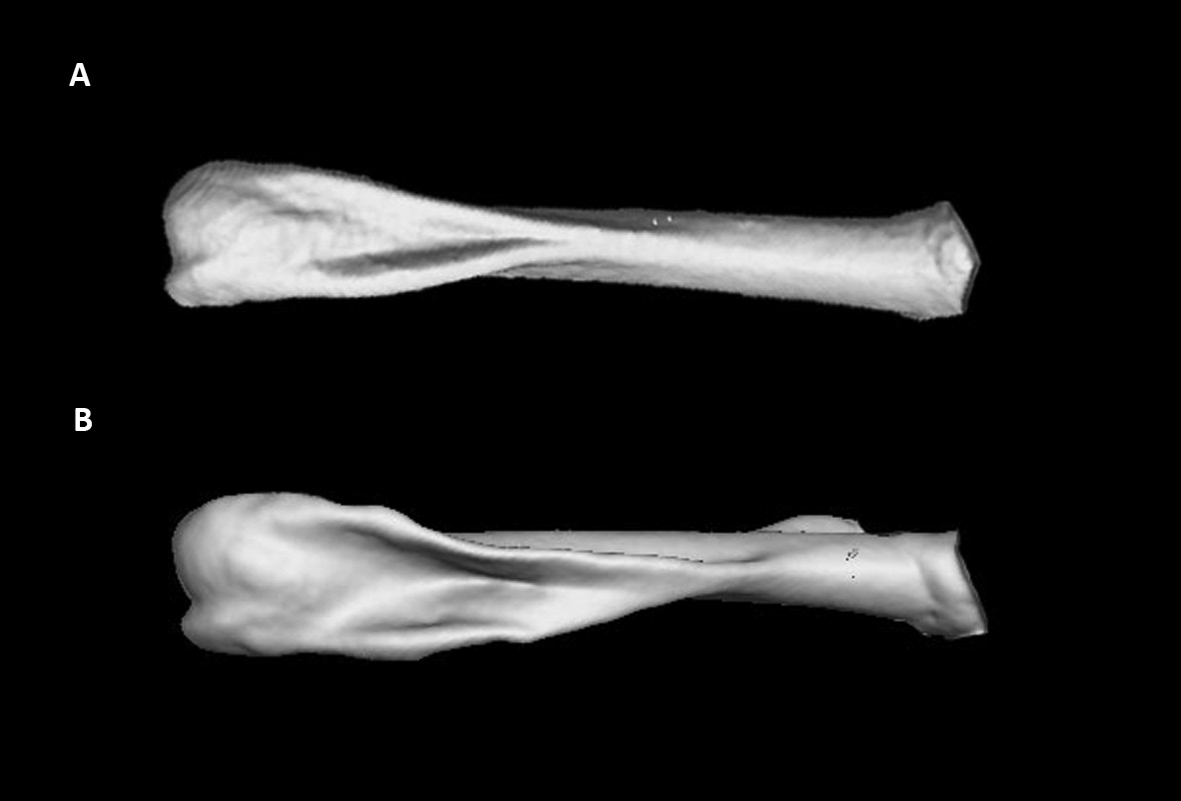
CT-scan image of the dorsal side of the humerus of *Petropedetes
